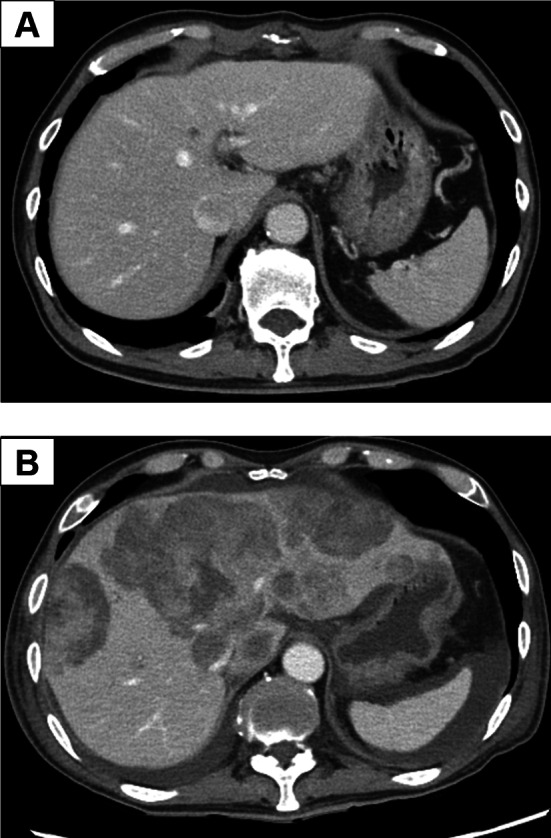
Postoperative enhanced abdominal computed tomography findings. A CT showing an 8 mm nodule on the hepatic segment 4 on POD 7. B Tumor in liver segment 4 increased to 85 mm on POD 41. Multiple intrahepatic liver metastases were also observed
